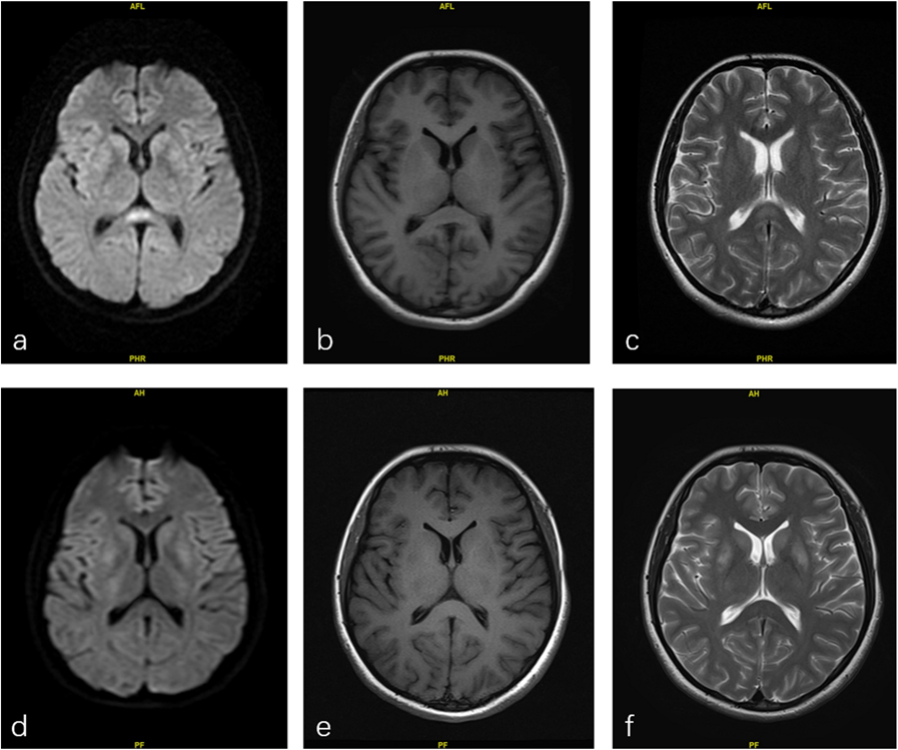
The lesion in the midline of SCC was hyperintensity on DWI a and T2WI c, isointense signals on T1WI b (2019-7-17). Follow-up (2019-8-9) images show complete resolution d, e,f)
